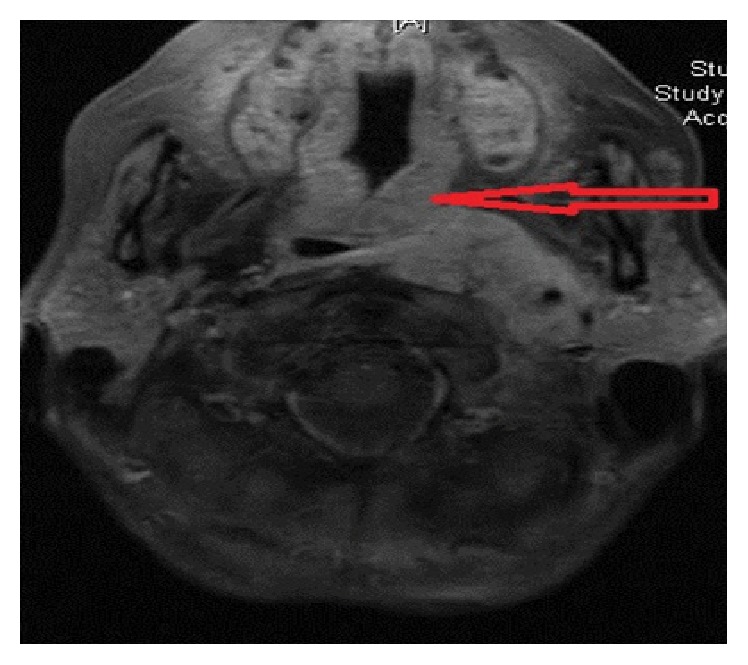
MRI of neck showing pharyngeal mass.
